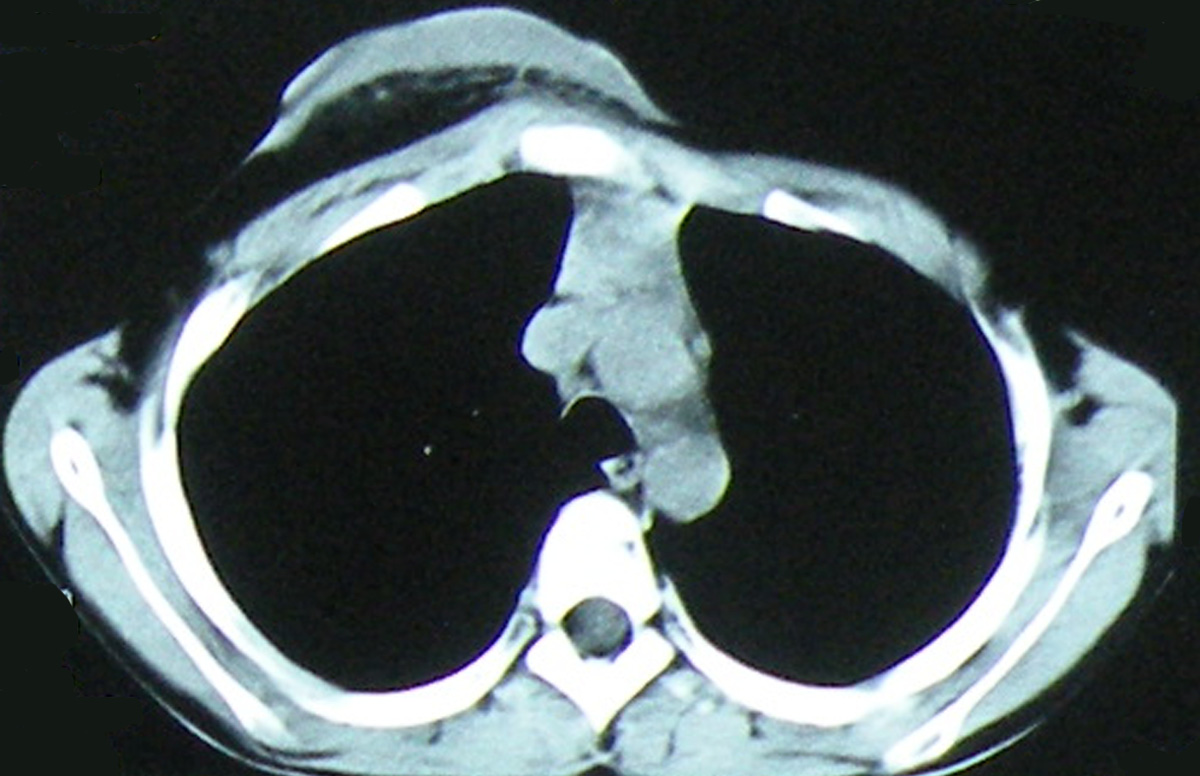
At the level of third thoracic vertebra, CT scan shows a well-define mass in the anterior chest wall without clear signs of infiltration of surrounding structures.
